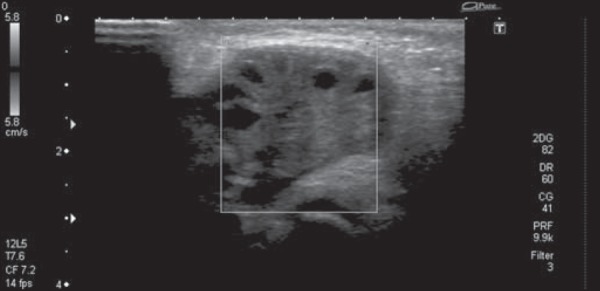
Doppler flow study showing the absence of vascular flow in the right
